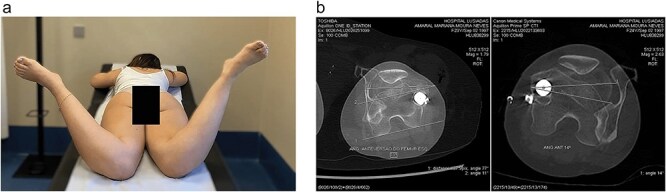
(a) Clinical examination showing hip internal rotation: 45° left and 45° right. (b) CT scans with measurement of both femoral versions correction.
